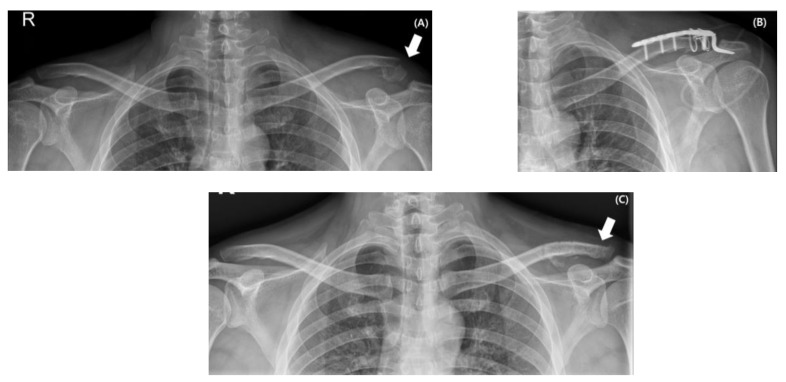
Serial radiographs of a 62-year-old man with a distal clavicle fracture (Type IID) sustained from a fall from height (indicated by a white arrow) (A). Surgical treatment involved hook plate fixation with additional cerclage wiring to assist in fracture reduction (B). Radiograph obtained at 1 year postoperatively confirmed maintained reduction and complete fracture healing (indicated by a white arrow) (C).
